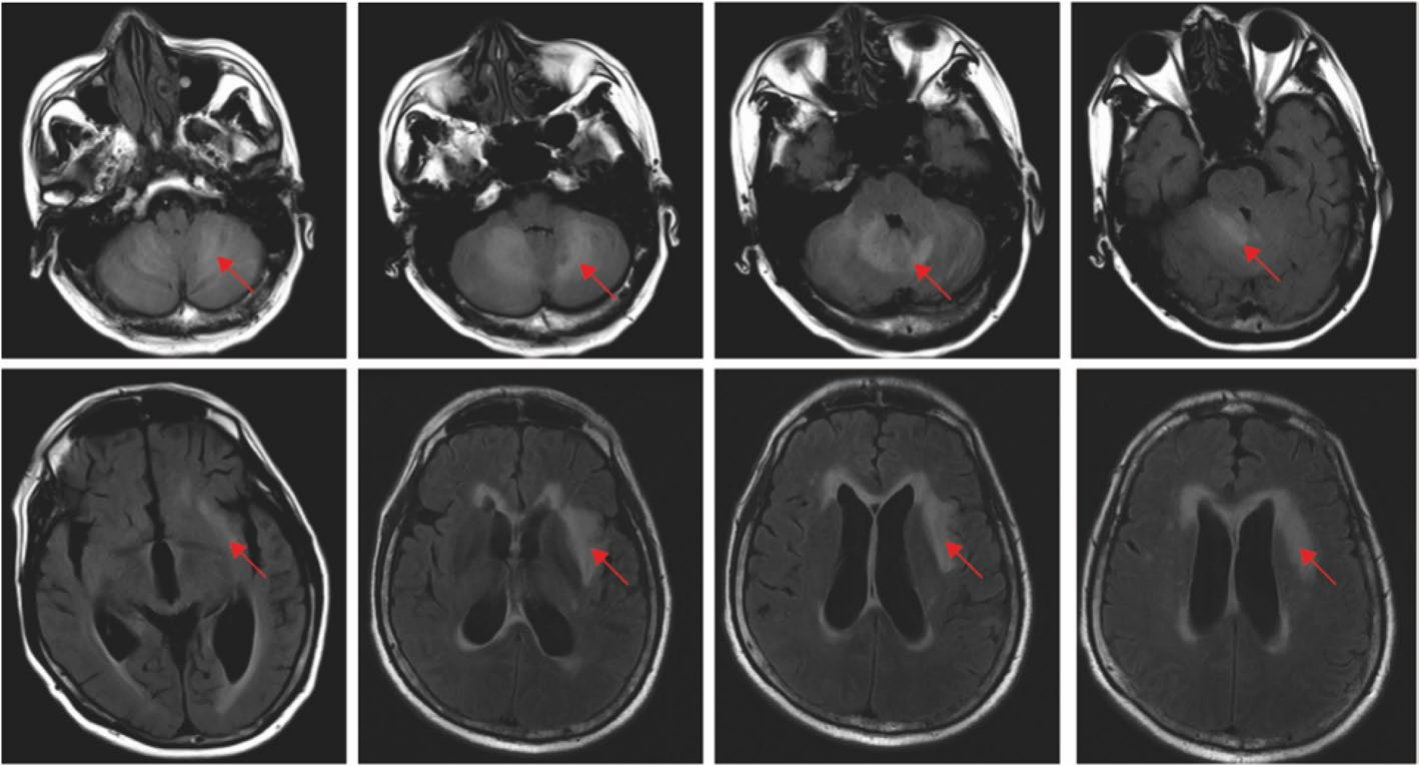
Plain head MRI: Abnormal signals in the left lateral capsule, insular lobe, and bilateral cerebellar hemispheres, with significant enhancement in the left insular nodule, left temporal nodule, and bilateral cerebellar meninges, with partial reduction of the focus than before. Arrowheads: infection lesions
